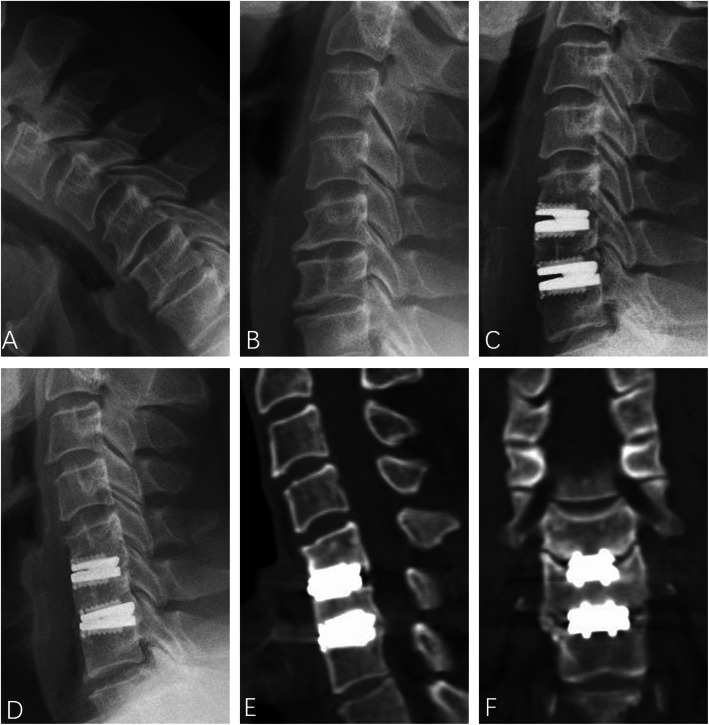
A 43-year-old male patient diagnosed with cervical spondylosis. The preoperative ROMs at C5/6 and C6/7 were 4.28° and 4.01°, respectively (a and b). The immediate postoperative X-ray (c) demonstrated the satisfactory location of Prestige-LP discs. The 87-month X-ray (d) and CT scans (e and f) showed the development of high-grade HO at C5/6 and C6/7
